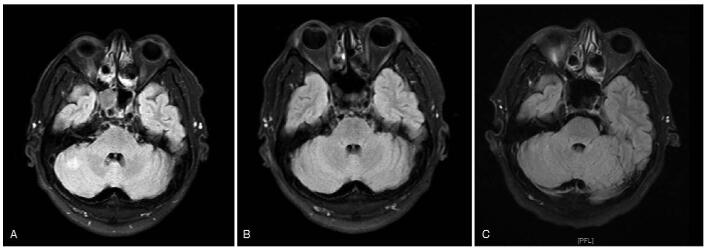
Head MRI imaging results for the case 1 during treatment with dacomitinib. (A) Brain MRI indicated the metastases in the right cerebellar hemisphere at diagnose (December 26, 2019). (B) The brain metastases were reduced prominently after 2 months of dacomitinib treatment, which was evaluated as a CR. (C) Head MRI showed no recurrence of BMs at the time of disease progresses (November 29, 2020). CR = complete response, MRI = magnetic resonance imaging
